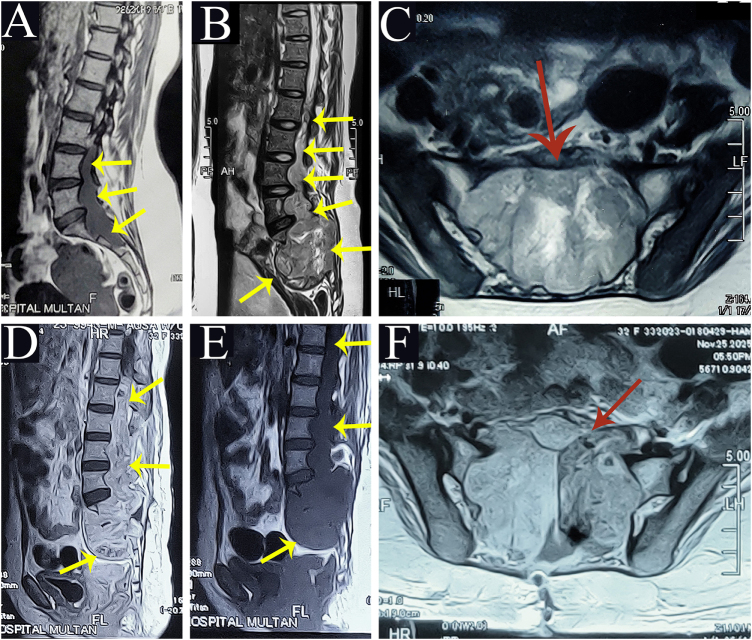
MRI of the lumbosacral spine demonstrating a giant myxopapillary ependymoma and postoperative status. (A) Sagittal T2-weighted non-contrast MRI showing an infiltrative lesion extending from L3 to S2. (B) Sagittal T2-weighted non-contrast MRI demonstrating marked progression with a large multilobulated mass extending from the conus medullaris (L1) to the sacrum. (C) Axial T2-weighted MRI showing sacral involvement and widening of the neural foramina. (D–E) Sagittal T1-weighted post-contrast MRI demonstrating decompression of the thecal sac with residual enhancing tumor. (F) Axial T1-weighted post-contrast MRI showing persistent sacral and foraminal involvement. Yellow arrows indicate tumor extent and thecal sac expansion, while red arrows highlight neural foraminal extension and sacral involvement.
